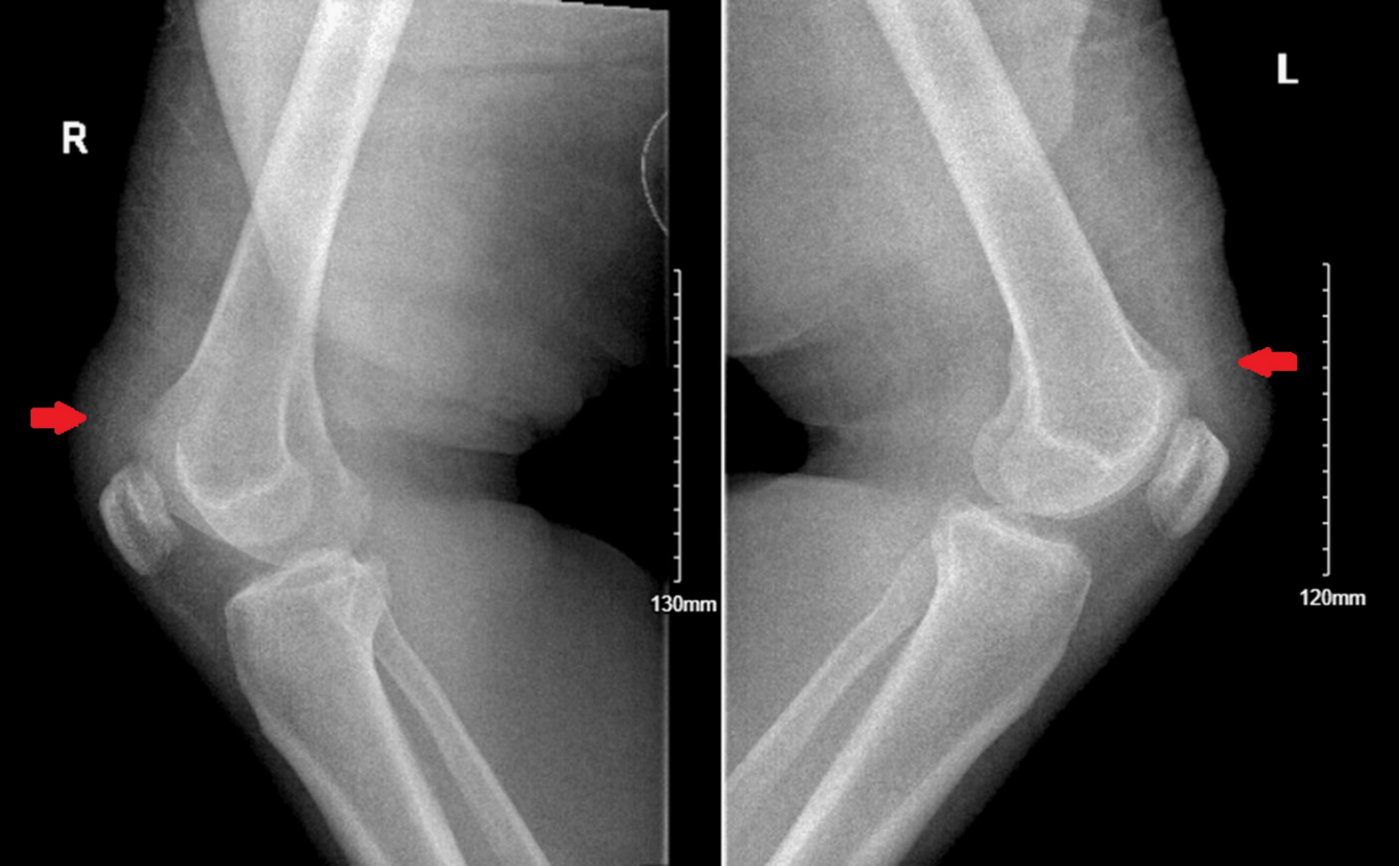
X-ray of bilateral knees in lateral view demonstrating small suprapatellar effusions
